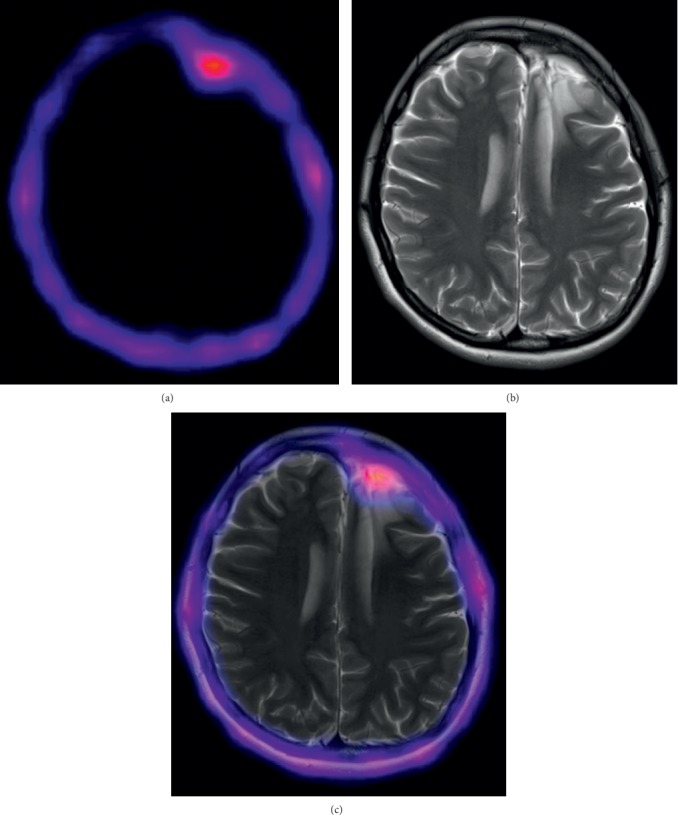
^99m^Tc-CXCR4-L SPECT (a), MRI (b), and SPECT/MRI (c) of a diffuse astrocytoma NOS (tumor recurrence) with *T*/*B*max 3.9 (patient 7).
